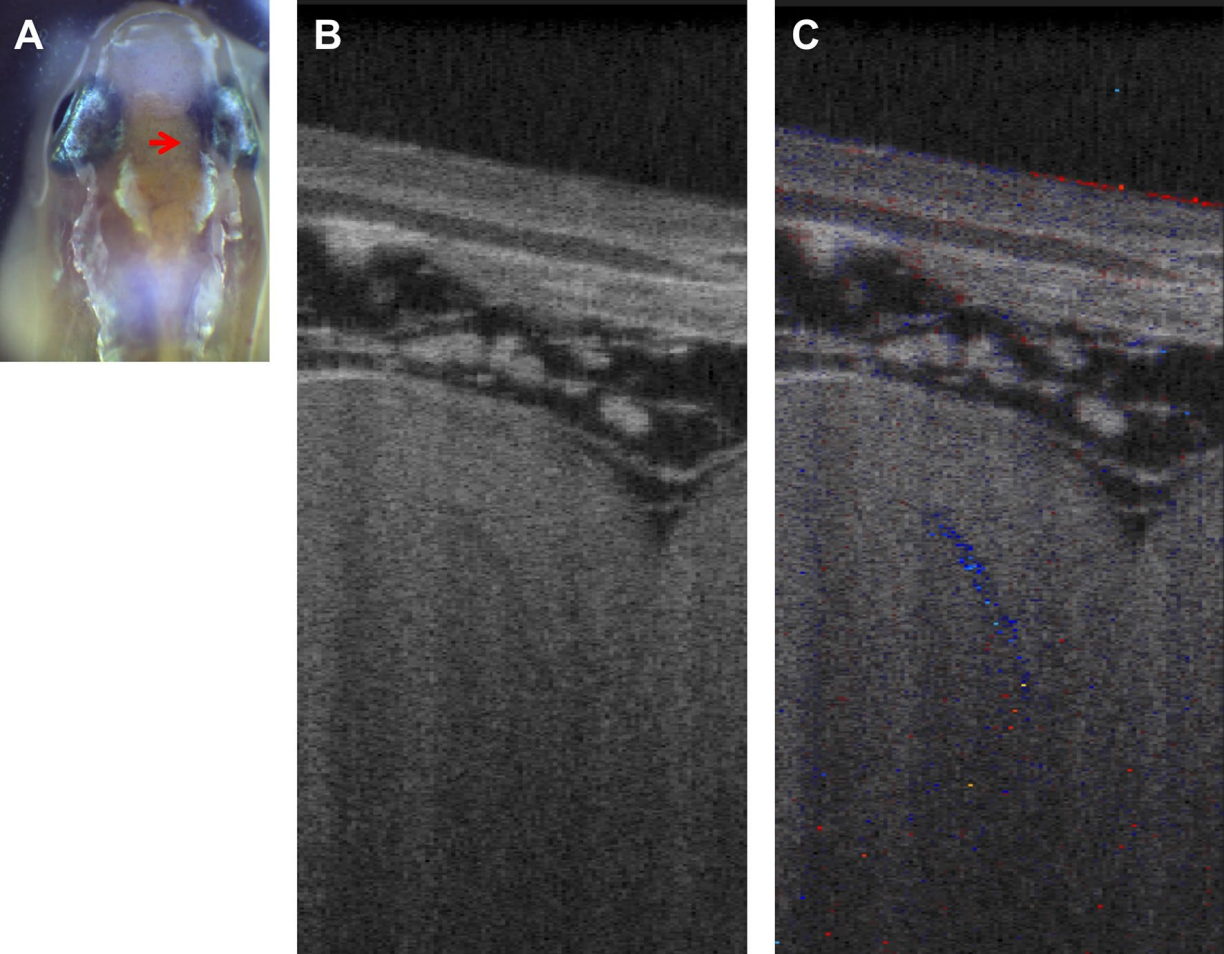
Verification of blood flow by Doppler OCT. (A) Video camera image (ThorImage®OCT v4.4, https://www.thorlabs.com/newgrouppage9.cfm?objectgroup_id=7982). The red arrow indicates the scan line and area (0.5 mm) of OCT. (B) 2D OCT image and (C) corresponding Doppler OCT image of the medaka telencephalon (ImageJ 1.52n, https://imagej.nih.gov/ij). The darker thread-like structure in (B) appears in blue in (C), indicating the presence of a Doppler frequency shift induced by a backward flow.
